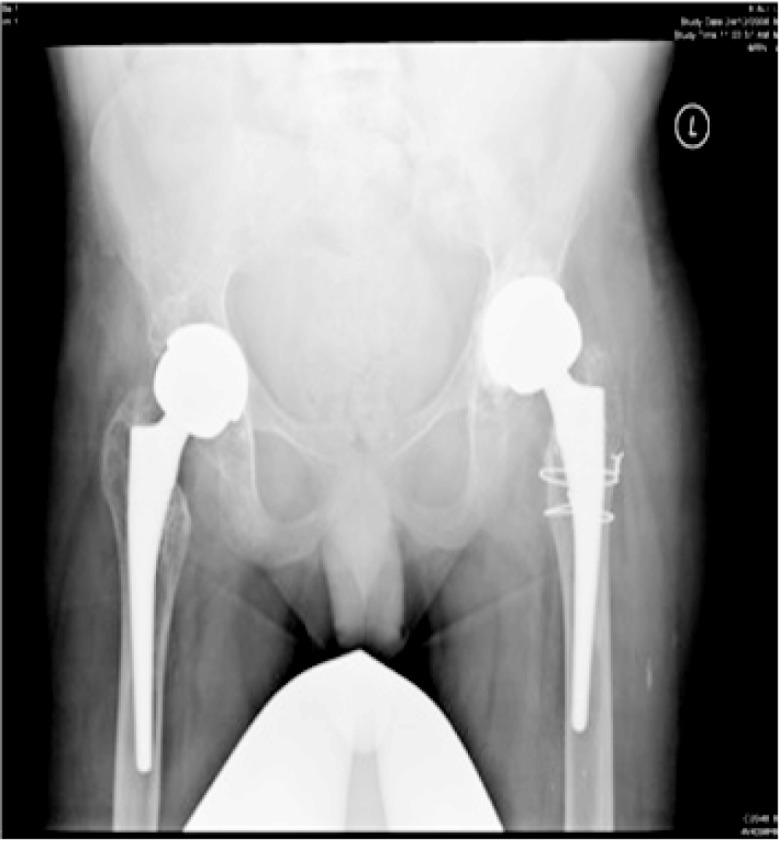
AP pelvis x-ray post bilateral THR.
